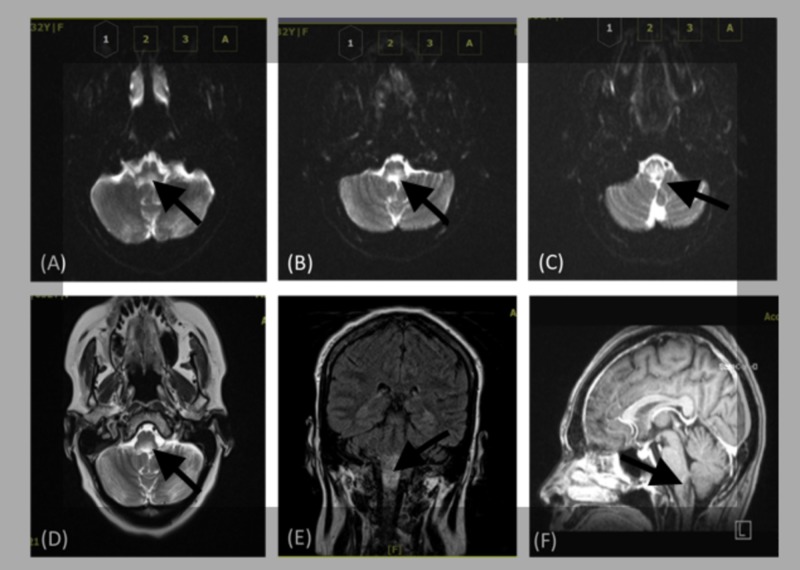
MRI T2 brain axial view with visualization of (see arrows corresponding to respective areas of interest) (A) lower medulla at the level of the cerebellum. Note a hyper-attenuated lesion situated at the dorsal medulla; (B) medulla and cerebellum at the lower maxillary level. Note the continuation of the hyper-attenuated lesion situated in accordance with the previous lesion, localized to the dorsal medulla; (C) medulla and cerebellum at the upper maxillary level. Note also the continuation of the hyper-attenuated lesion situated in accordance with the previous lesion, localized to the dorsal medulla; (D) MRI T2 FLAIR brain axial view with visualization of the lower medulla at the level of the cerebellum. Note a hyper-attenuated lesion situated at the dorsal medulla with increased T2 signal and mild diffuse and heterogeneous enhancement; (E) MRI T1 brain coronal view depicting a 1 x 2.5-centimeter hyper-attenuated, isolated lesion at the dorsal medulla. (F) MRI T1 brain sagittal view depicting a 1 x 2.5-centimeter isolated lesion at the dorsal medulla. There was no definite enlargement of the bones or the upper cervical cord. There were no definite areas of abnormal enhancement. There were no areas of restricted diffusion. MRI: magnetic resonance imaging; FLAIR: fluid-attenuated inversion recovery
